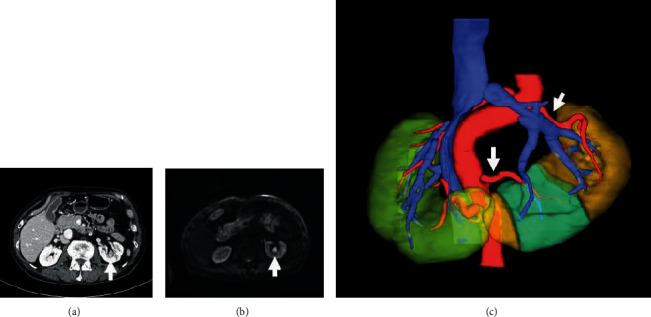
(a) CT findings. CT reveals a mass lesion of upper pelvis of the left side of the horseshoe kidney (arrow). (b) MRI findings. MRI reveals a high signal intensity on diffusion-weighted imaging (arrow). (c) Reconstruction of three-dimensional analysis of the images of thin-slice contrast-enhanced CT. Three-dimensional reconstruction reveals two renal arteries arising from the aorta that supplied to the left side of the horseshoe kidney (arrow).
